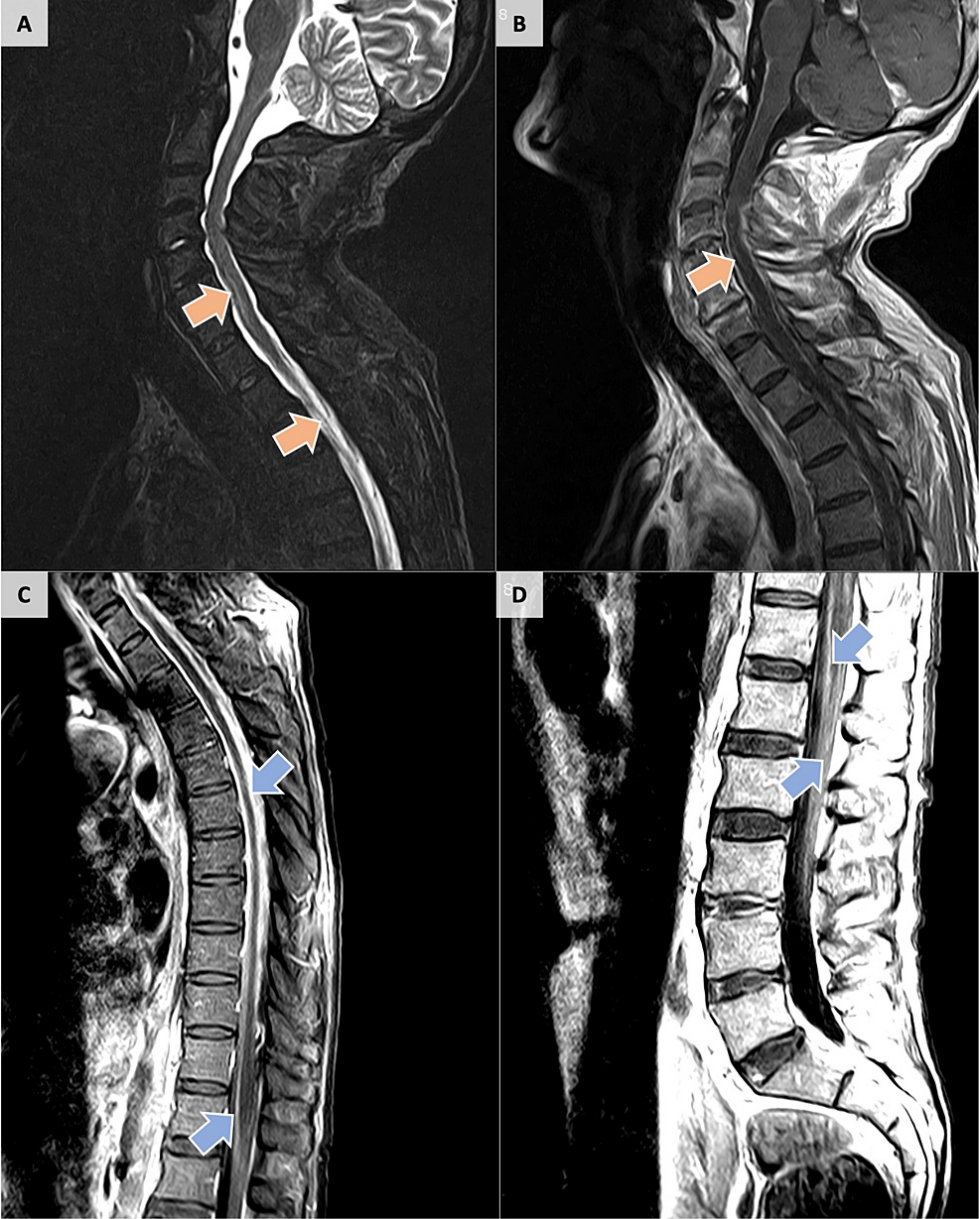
Magnetic Resonance Imaging of spinal cord (A) Sagittal T2 STIR and (B) Sagittal T1 GAD: high T2 signal in the anterior aspect of cervical and thoracic spinal cord, with multifocal T1 gadolinium enhancement. (C) Sagittal T1 GAD fatsat thoracic spine and (D) Sagittal T1 GAD lumbar spine: pachymeningeal and leptomeningeal T1 gadolinium enhancement from C2/3 to cauda equina. STIR: short tau inversion recovery; GAD: gadolinium contrast; fatsat: fat-saturation
